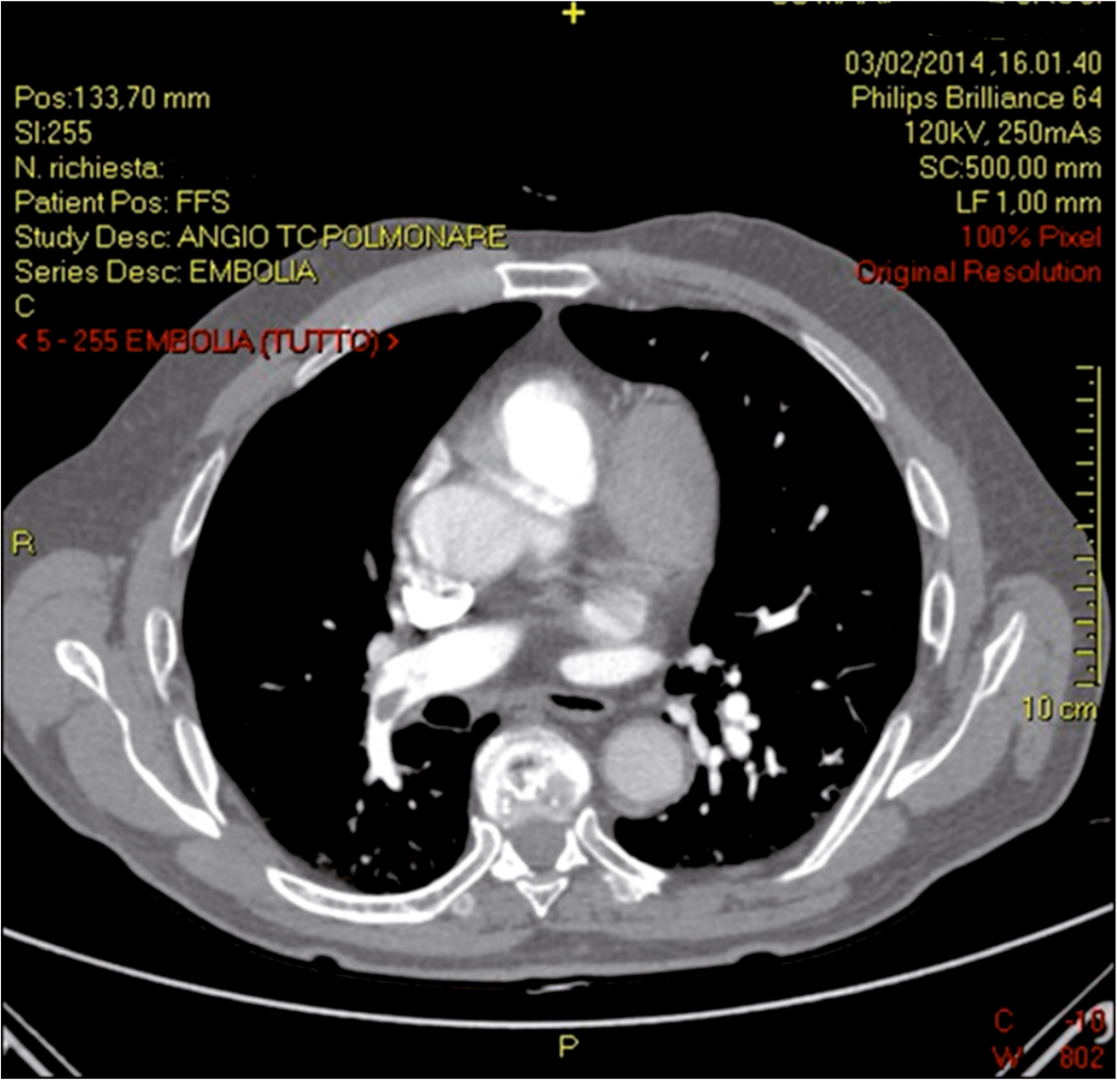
Computed tomographic pulmonary angiography at the 7-day follow-up: partial reperfusion of lower lobe pulmonary arteries. The other computed tomography findings appear unchanged
